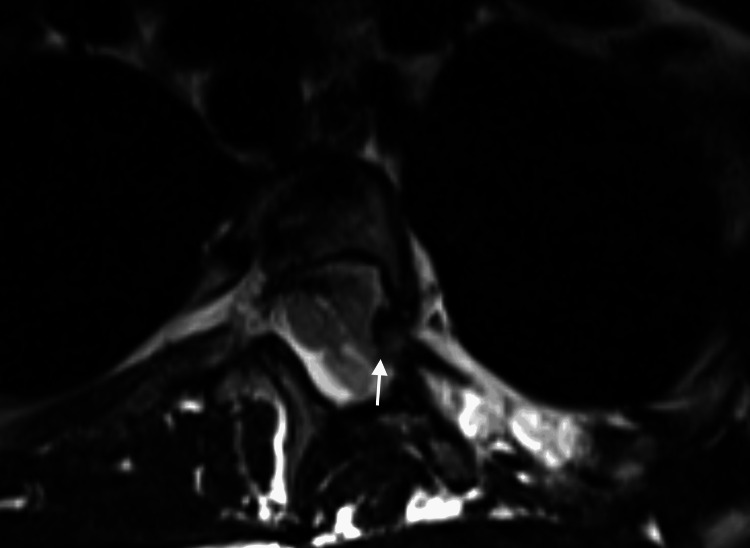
Axial T2-weighted image through the T4-T5 level showing protrusion of the left T5 rib head through the neural foramen into the spinal canal (arrow). There is no cord compression or signal abnormality at this level. Note extensive CSF pulsation artifacts within the thecal sac.
